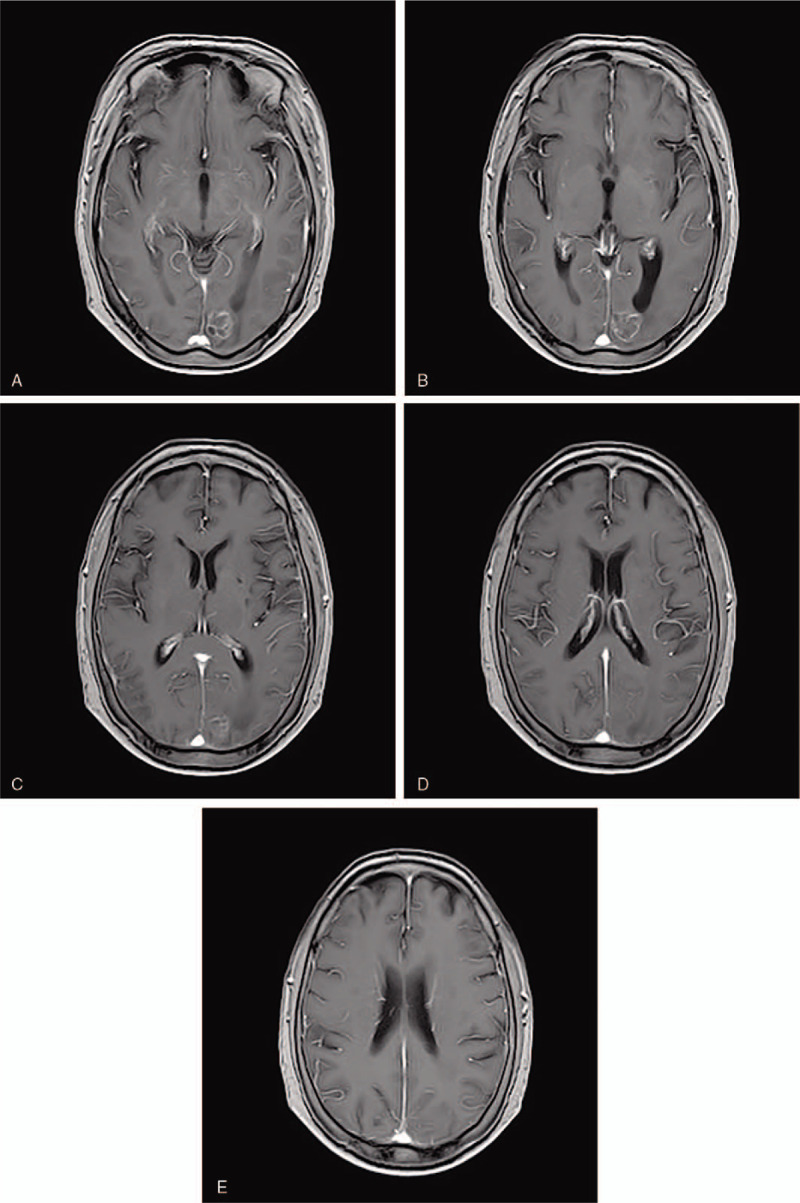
(A–E) Magnetic resonance imaging of left occipital metastasis 2 mo after radiotherapy.
